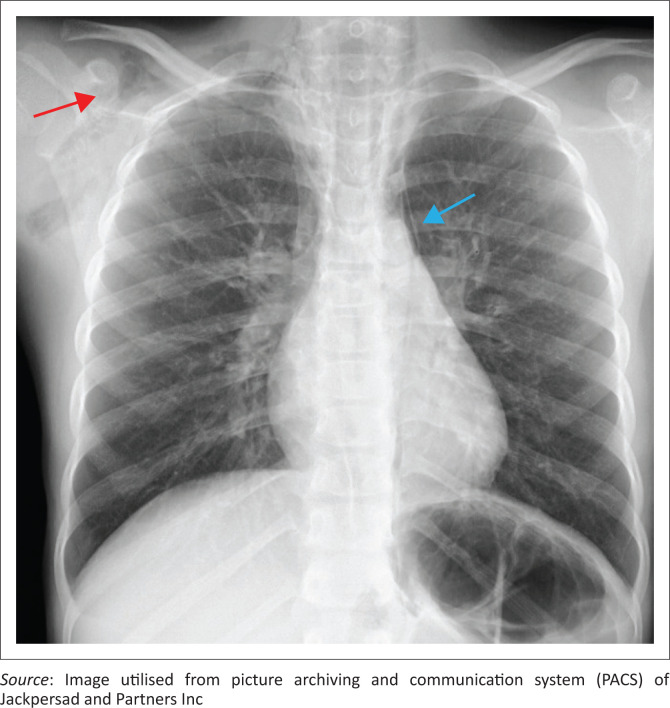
Chest radiograph on admission demonstrating subcutaneous emphysema (red arrow) and pneumomediastinum (blue arrow).
